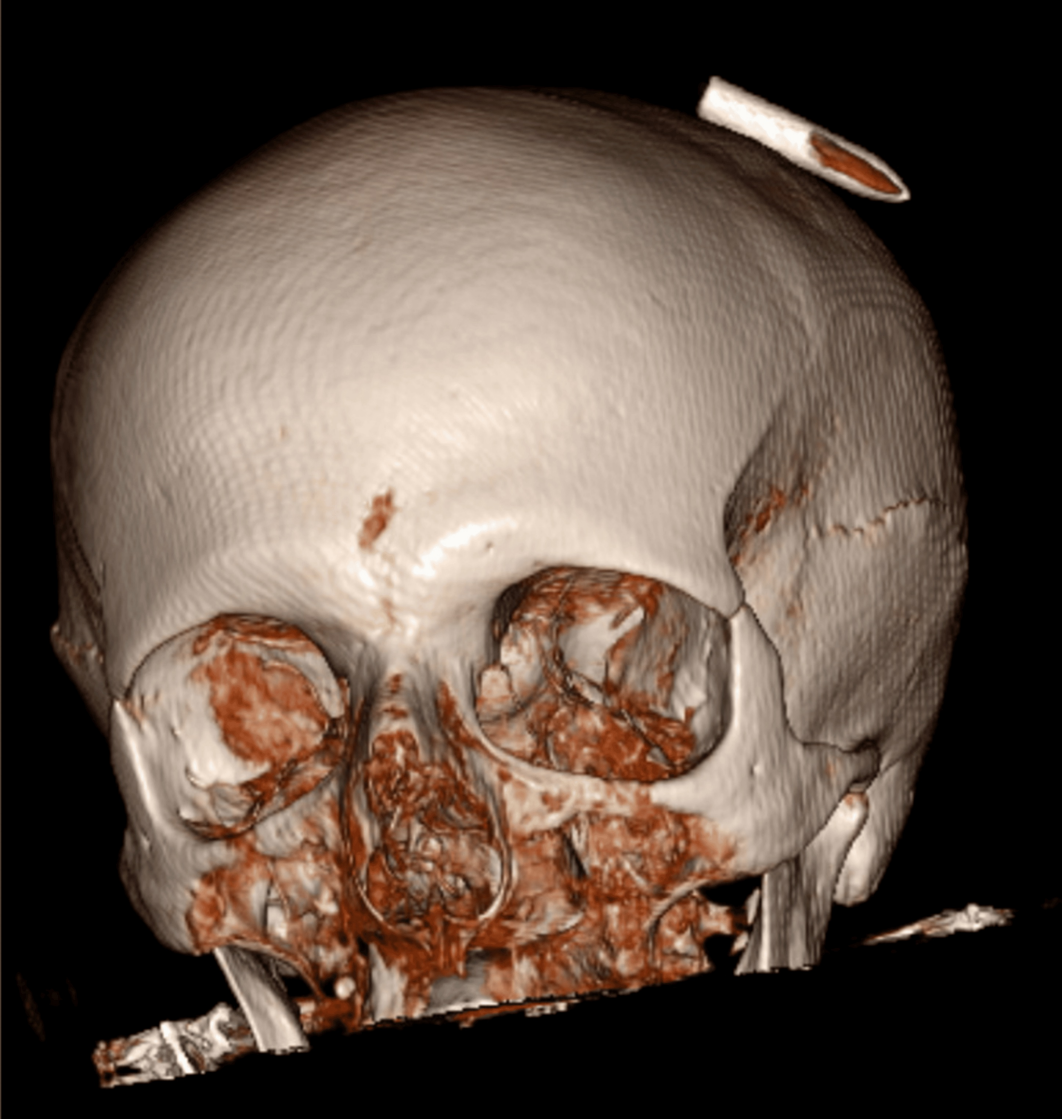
3D head CT reconstruction. The arrow tip is positioned just above the external cortical bone, with no evidence of intracranial involvement. CT: computed tomography
